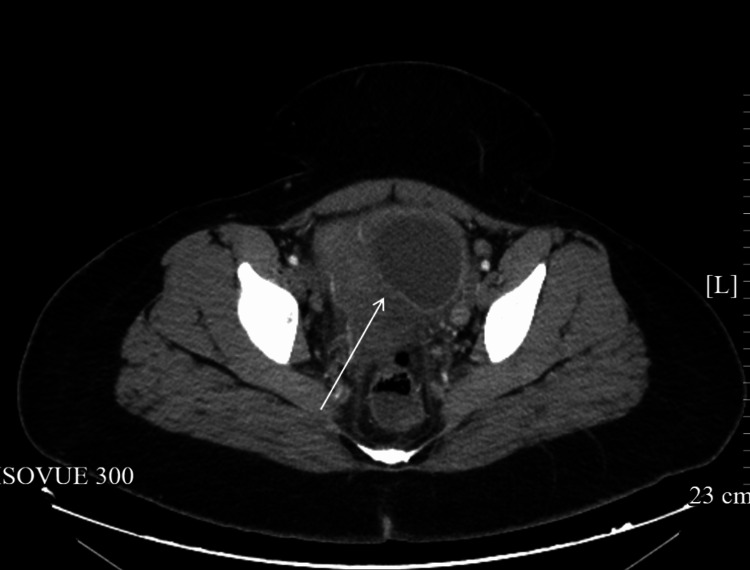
CT with contrast revealing a large cyst consistent with a tubo-ovarian abscess. The arrow points toward the large cyst.
